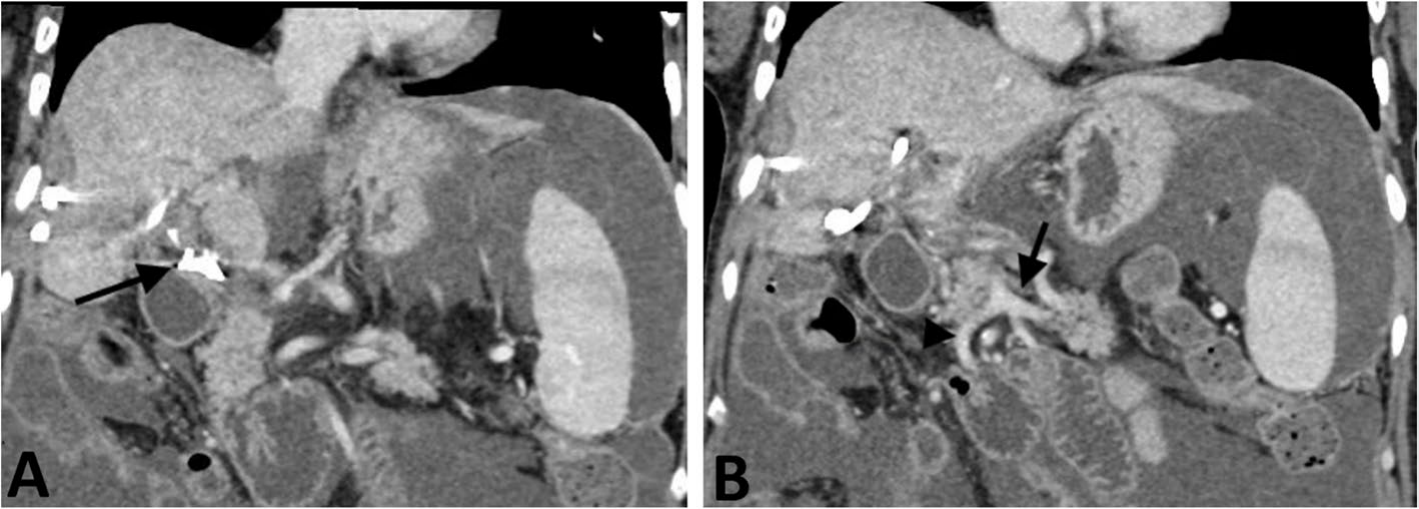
A Coronal CT of the abdomen demonstrates the site of portal vein ligation (black arrow) without distal filling of portal vein branches. B Coronal CT of the abdomen demonstrates patient splenic vein (black arrow) and superior mesenteric vein (black arrowhead)
